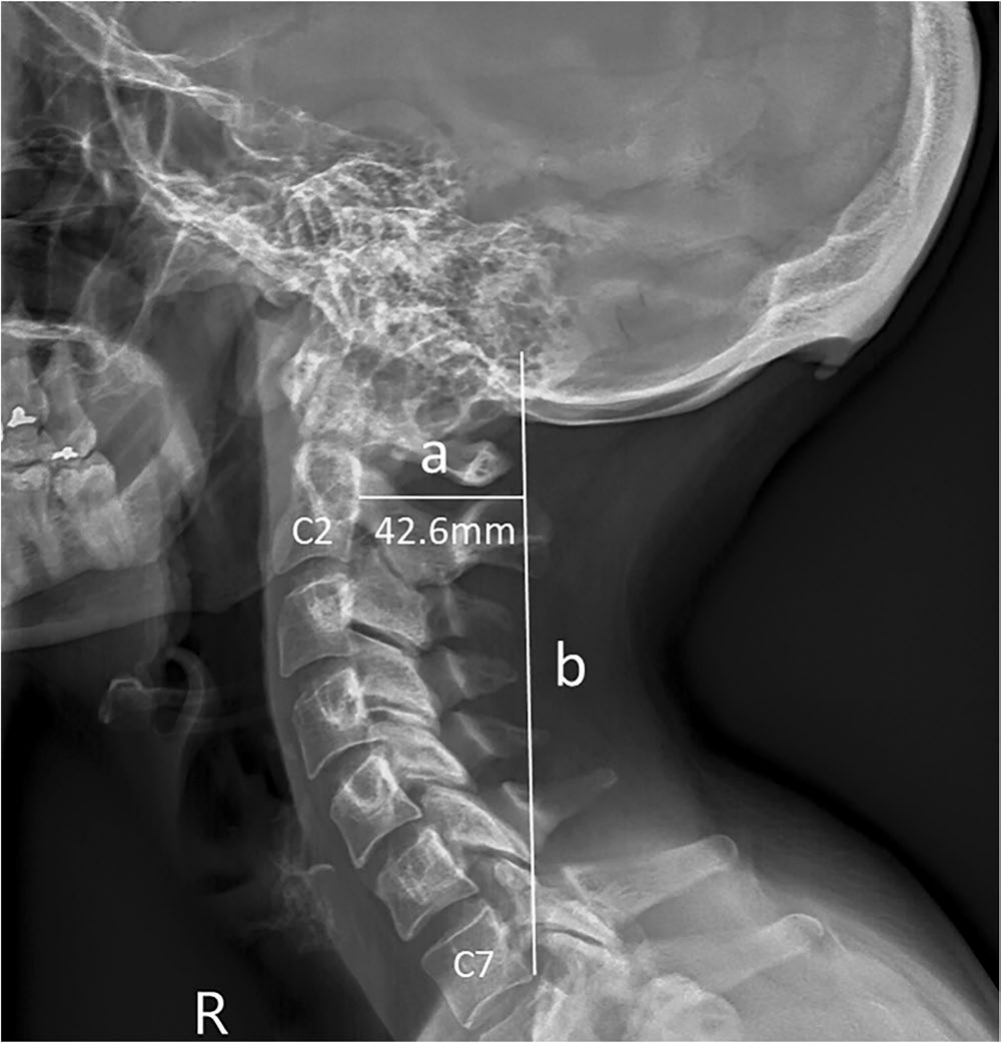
Sample radiographic assessment of forward head protraction (FHP). The extent of FHP was determined by measuring the length (in millimeters) of a horizontal line (a) drawn from the margin of the posterior-superior corner of the body of C2, to a vertical line (b) drawn up from the margin of the posterior-inferior corner of the body of C7 on a lateral cervical radiograph^23^.
